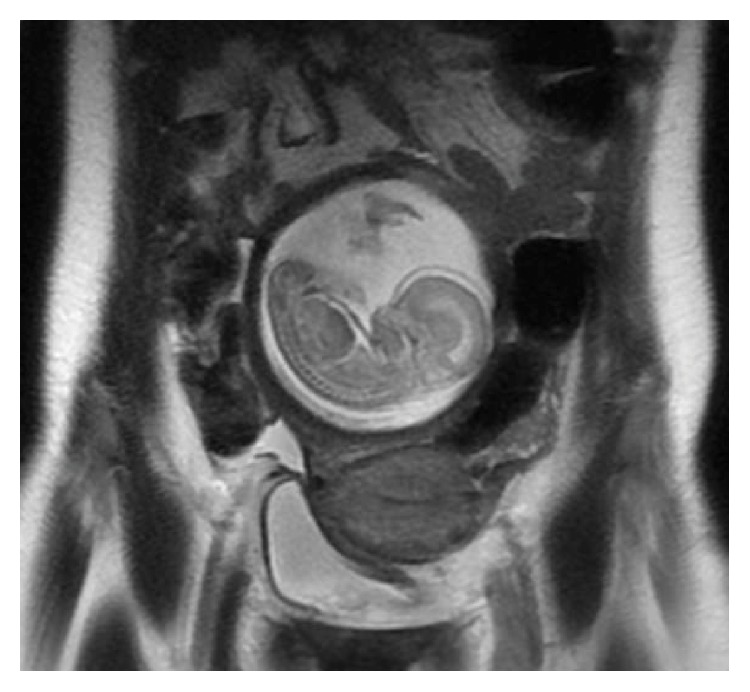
Preoperative MRI showing fetus in right uterine horn with no visible channel between left and right uterine horn.
